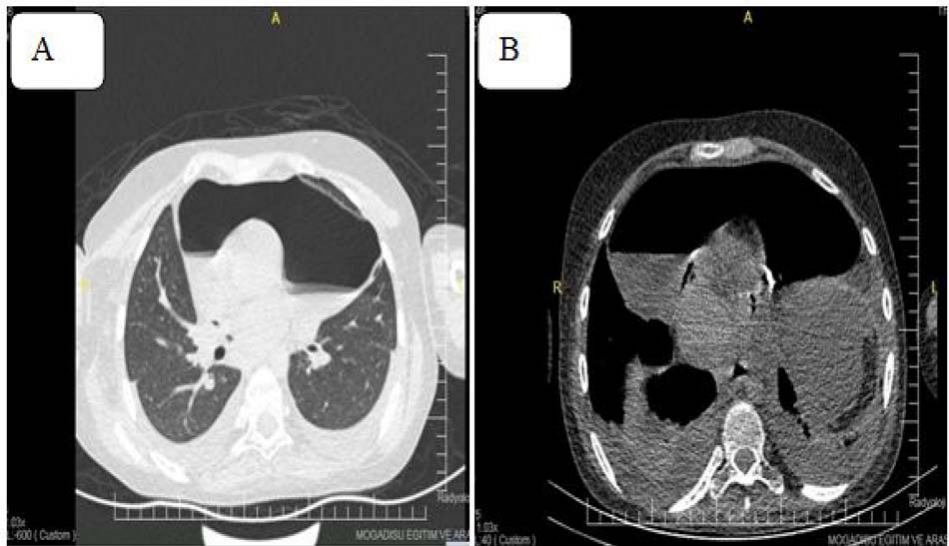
Pneumoprecadium on chest CT (A) and moderate pleural effusion (B).
